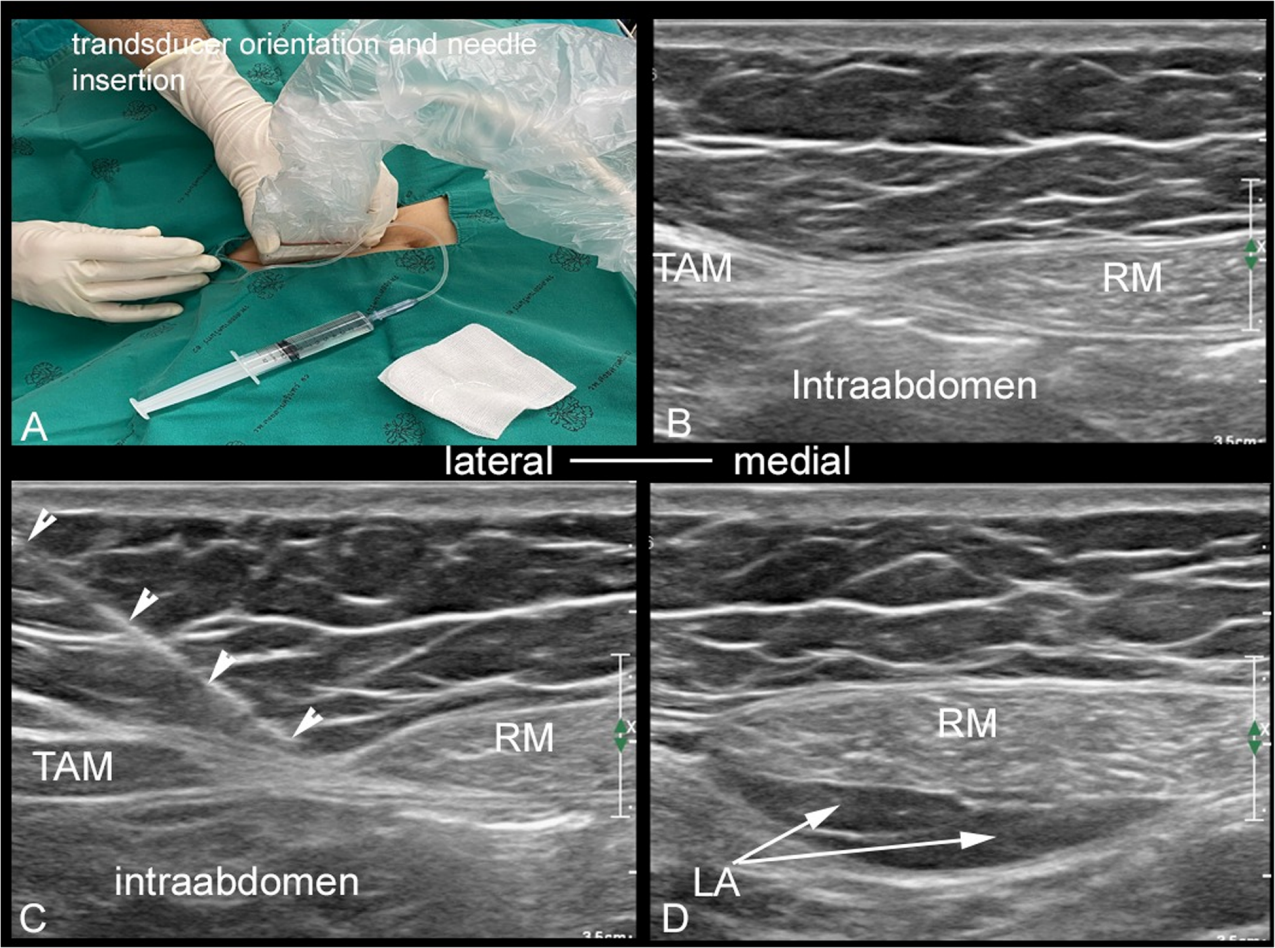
Ultrasound-guided rectus sheath block technique. *RM* rectus sheath muscle, *TAM* transversus abdominis muscle
